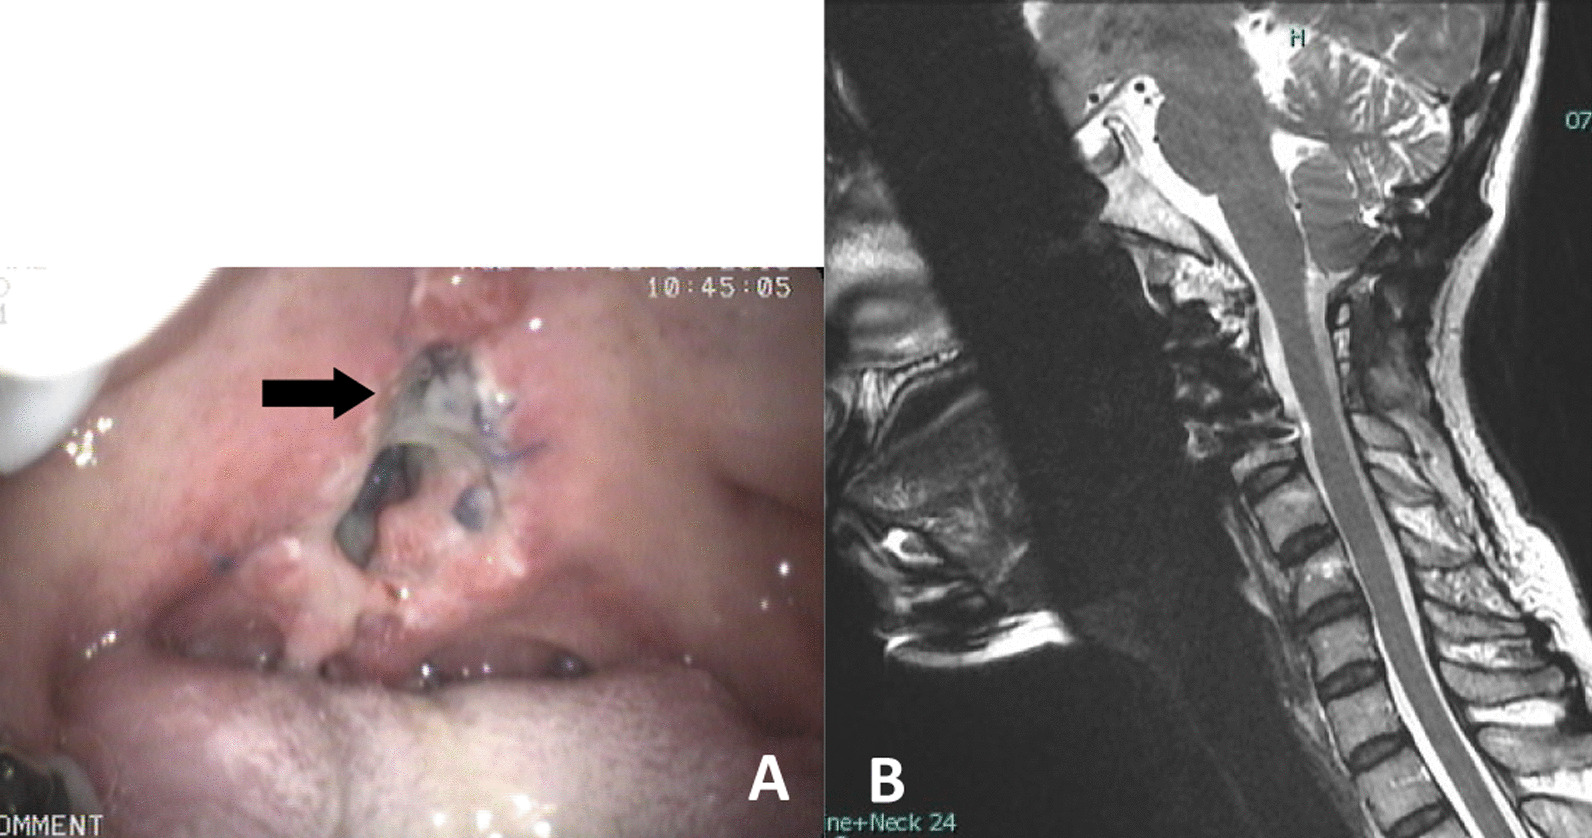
Surgical wound dehiscence and the exposed anterior cervical plate (black arrow) were observed before radiotherapy (A). Midterm (5 year) follow-up magnetic resonance imaging of the cervical spine revealed no gross tumor recurrence at the surgical bed (B)
